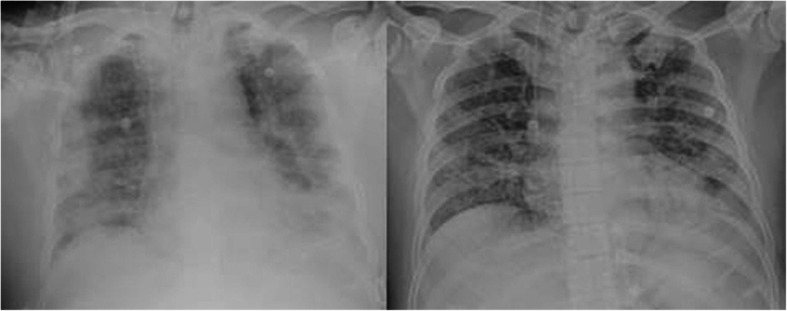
Portable chest X-rays of our COVID-19 patient depicting interstitial infiltrates and consolidations (day-1) prior to therapeutic plasma exchange (TPE) (*left panel*); and after five sessions of TPE (day-5) showing gradual improvement (*right panel*)
